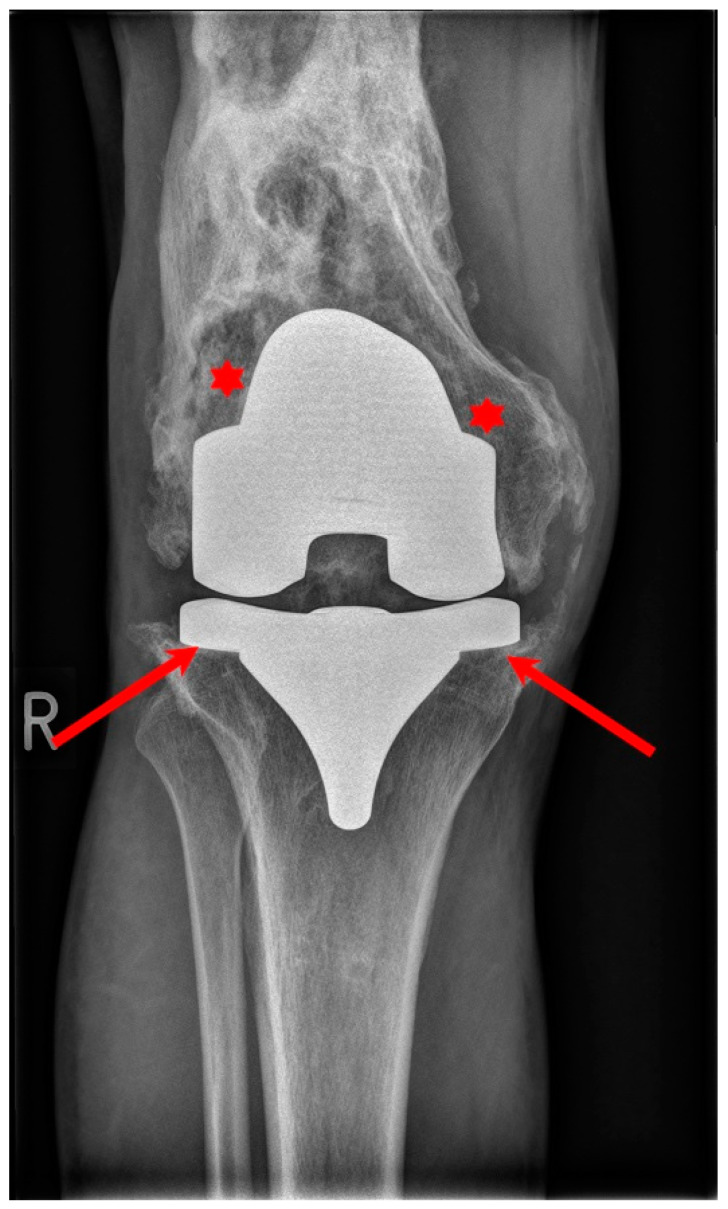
Conventional X-ray showing septic loosening of the TKR with bone destruction (asterisk) and loosening of the implants (arrows).
